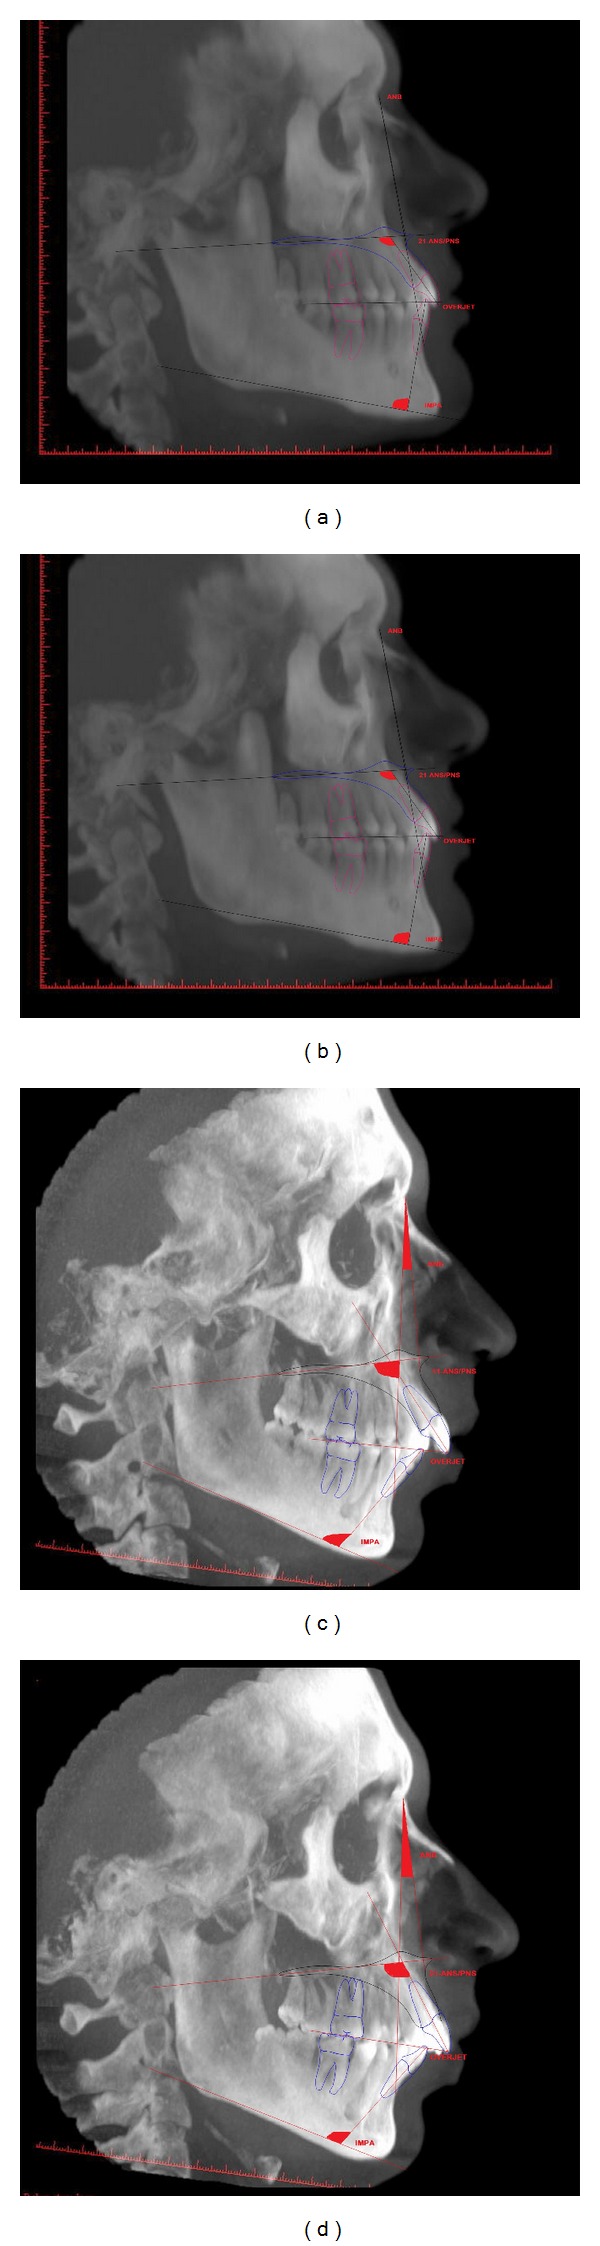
Examples of CBCT right and left of two subjects. The first two figures belong to a subject with ANB 0°–4° (skeletal class I), the second two to a subject with ANB >4° (skeletal class II). In the images, axes and angles like ANB, IMPA, U1-bispinal plane, and overjet were drawn to explain the aim of this work. In the research, each parameter was analysed via NNT NewTom 3G software using particularly secondary reconstruction.
